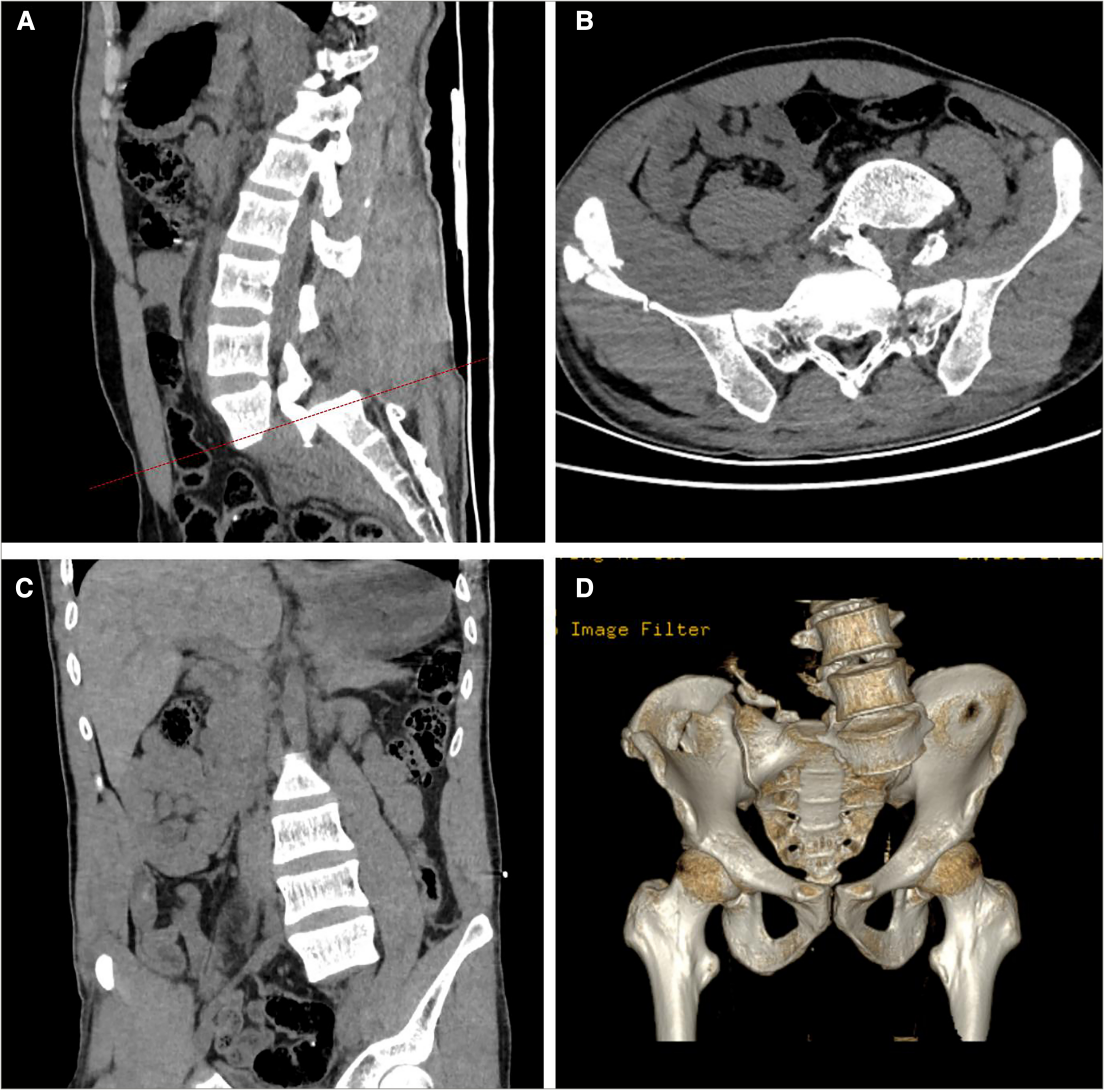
Sagittal (A), cross-sectional (B), and coronal (C) CT images showed the patient was definitely diagnosed with lumbosacral spondyloptosis. The three-dimensional reconstruction was useful in showing the bony structure clearly (D). CT, computed tomography.
